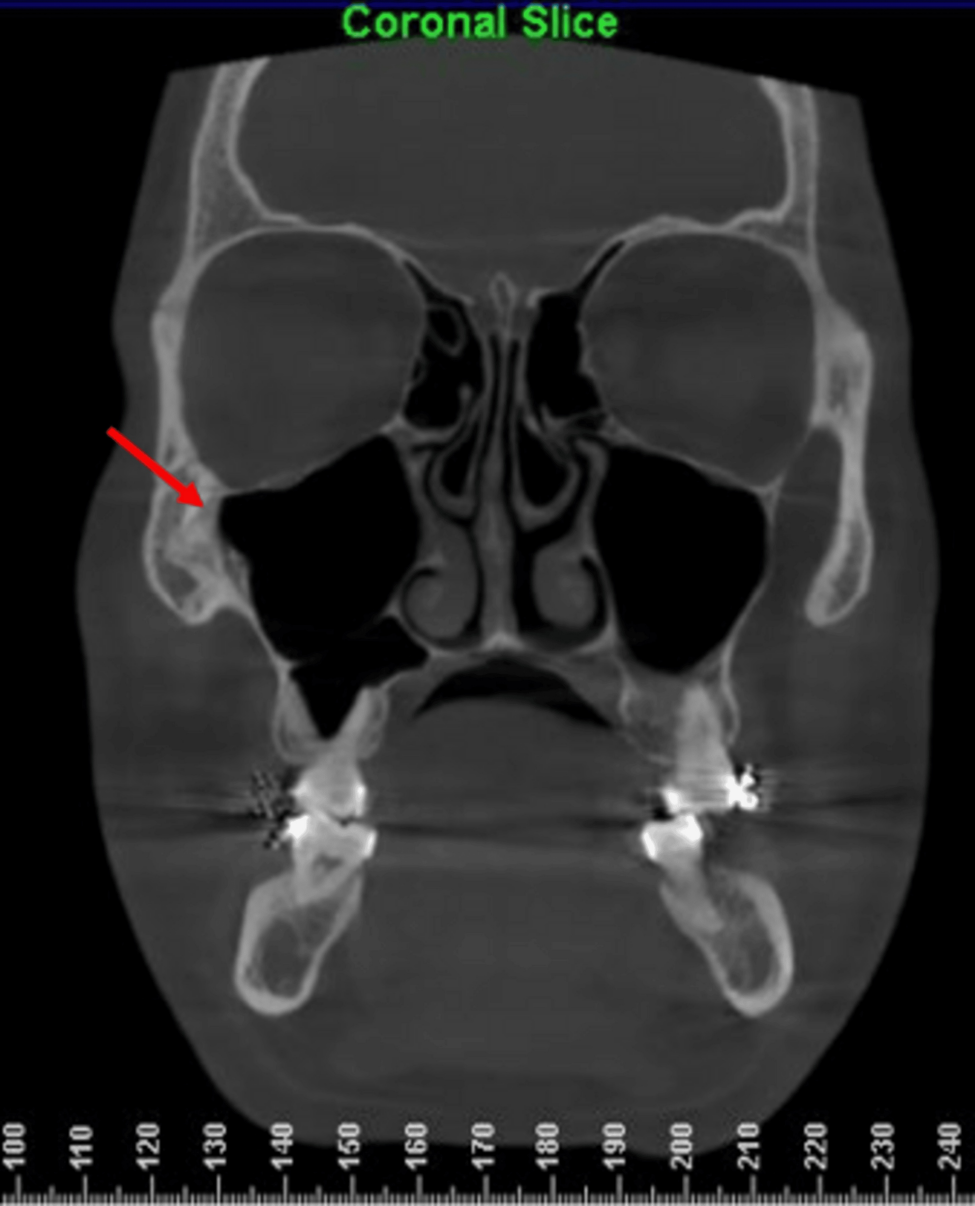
Coronal slice on a CBCT showing the exact location of the zygomaticomaxillary suture. The red arrows indicate the exact location of the suture in all coronal sections. CBCT: Cone-beam computed tomography.
